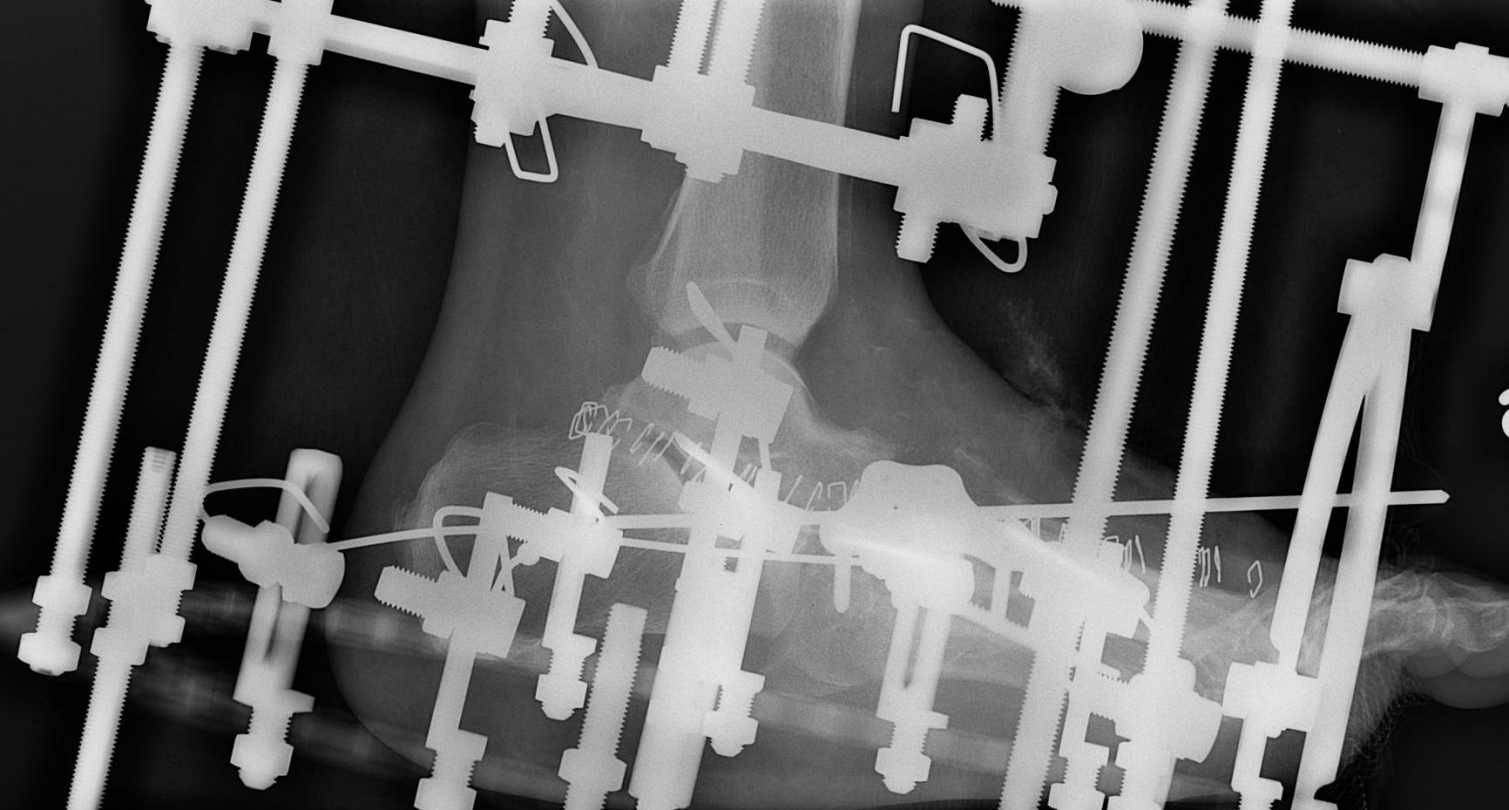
Lateral radiographic views showing the multiple midfoot arthrodesis sites with combined internal and external fixation methods.
